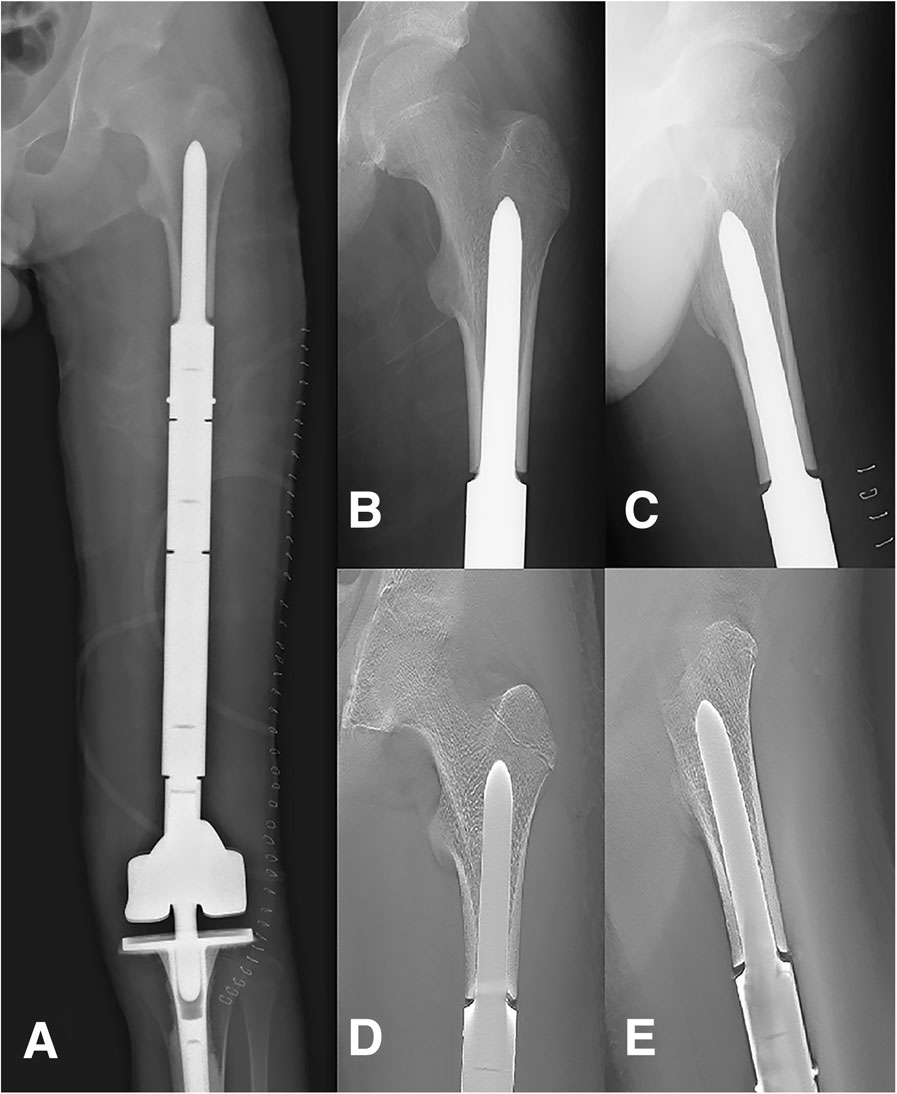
A case of reconstruction of the distal femur following distal resection of 73% of the length of the femur. a Postero-anterior radiograph of the entire femur. b, c Postero-anterior and lateral radiographic views of the region of stem insertion of the femur. d, e Postero-anterior and lateral T-SMART views of the stem insertion region of the femur
